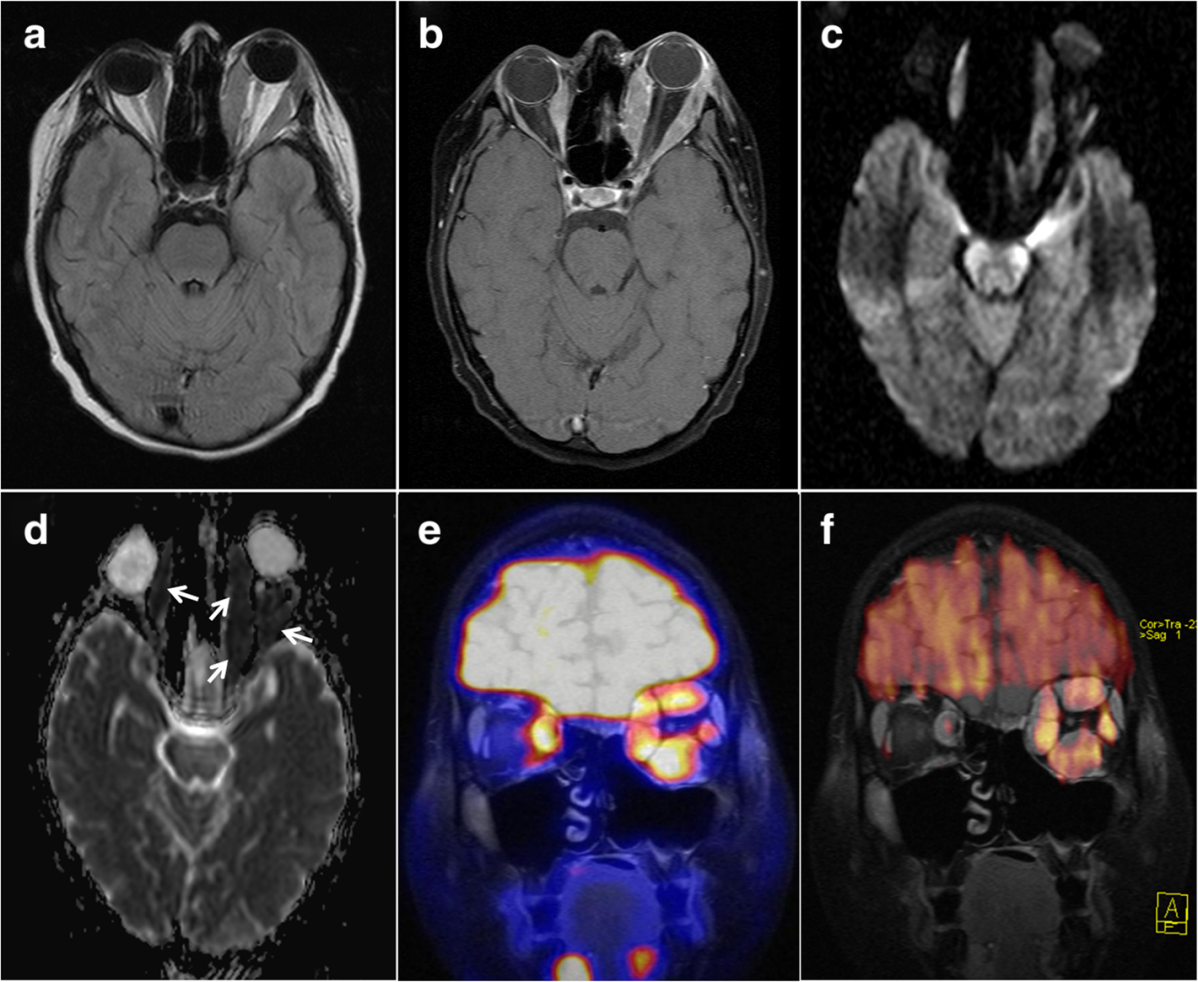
Rhabdomyosarcoma metastases to the extraocular eye muscles. Excessive bilateral thickening of extraocular eye muscles and left-sided exophthalmos two months after onset of symptoms. (a) pre-contrast T1w, (b) contrast-enhanced fat-saturated T1w, (c) DWI b = 1000 s/mm^2^ and (d) ADC map indicating markedly restricted diffusivity in the extraocular eye muscles (arrows), fusion of MRI (coronal contrast-enhanced fat-saturated T1w) with FDG-PET (e) and with colour-encoded DWI b = 1000 s/mm^2^(f).
